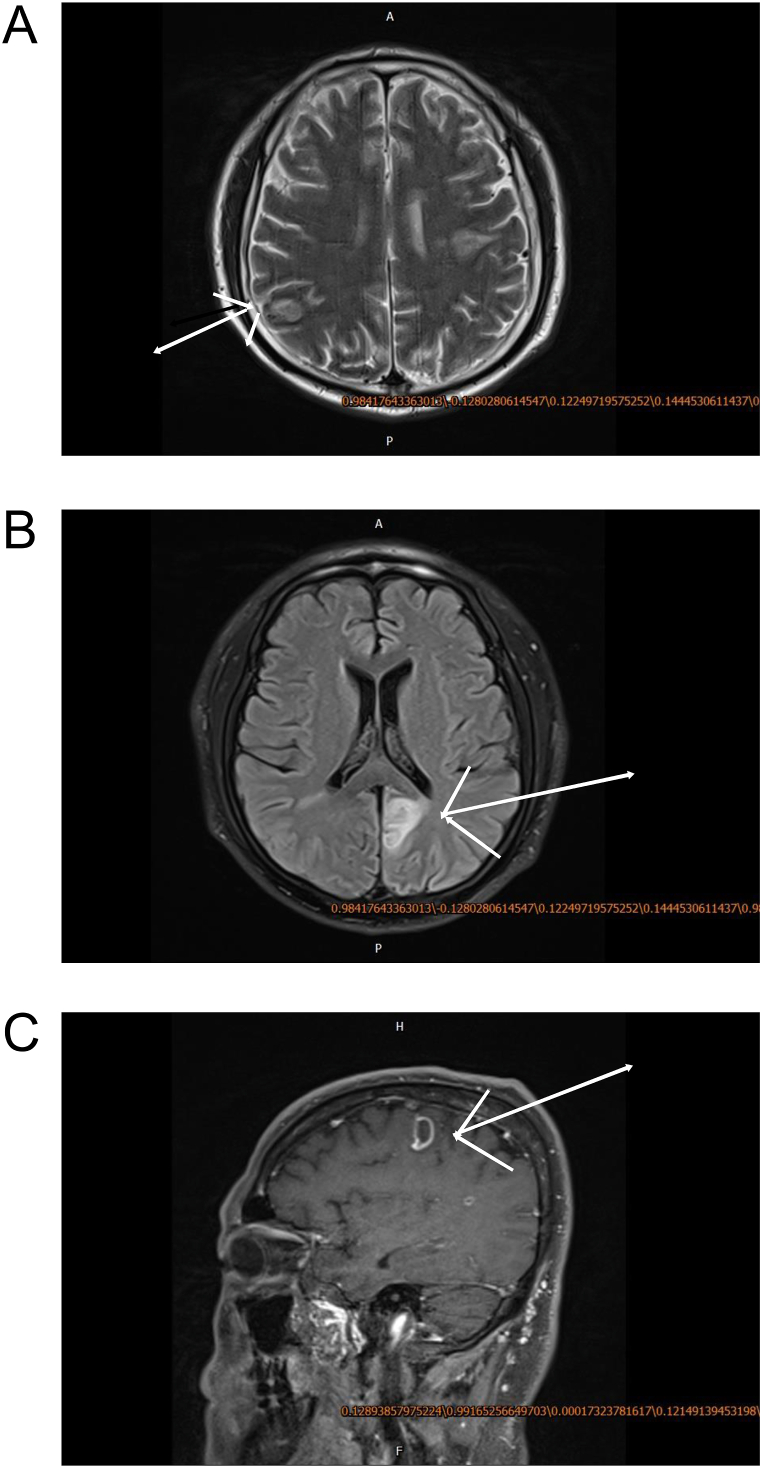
Magnetic resonance imaging of the head with multiple ring-enhanced abscesses. A. Axial position. B. Coronal position. C. Sagittal position.
